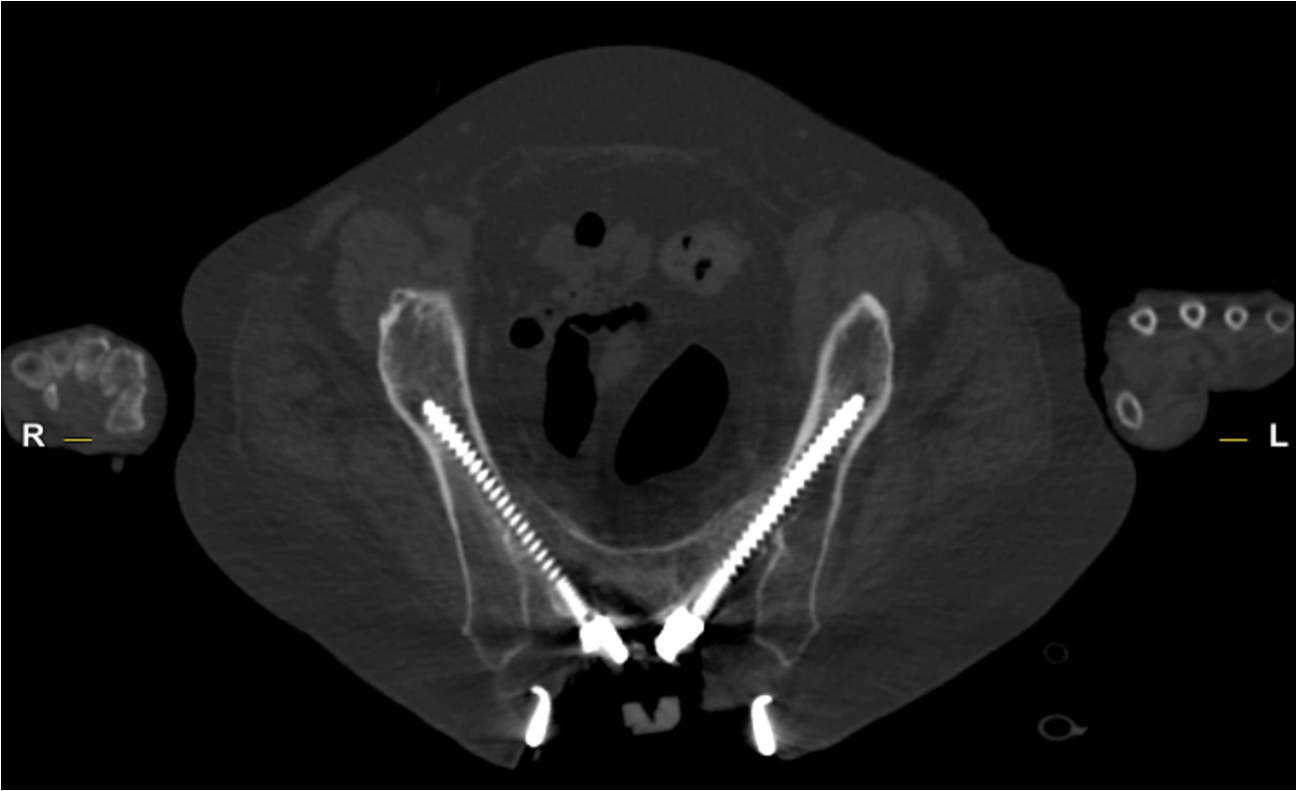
S2-alar-iliac technique. This slide shows the axial computed tomography (CT) slice of bilateral S2-alar-iliac (S2AI) screws. The entry point is between the dorsal S1 and S2 foramens. The screw then crosses the sacroiliac joint into the pelvis above the iliac notch via a trajectory targeting the head of the femur
